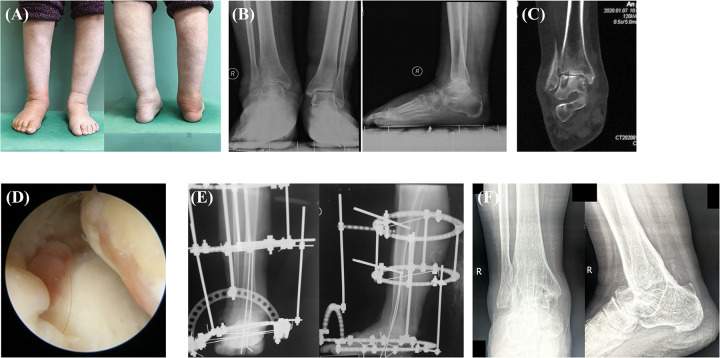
A 75-year-old female patient presented with a three-year history of inexplicable swelling and pain in the right ankle joint, with exacerbation over the past year characterized by swelling, warmth, and nocturnal pain (A). Preoperative x-ray (B) and CT (C) imaging revealed joint damage and arthritic changes in the ankle and subtalar joints. Arthroscopy-assisted right tibiotalocalcaneal debridement and arthrodesis was performed. We found the cartilage detachment and synovial hyperplasia during surgery (D). Postoperative x-ray assessment demonstrated favorable fusion positioning and proper hindfoot alignment (E) At final follow-up, the satisfactory osseous consolidation and hindfoot alignment were observed (F).
